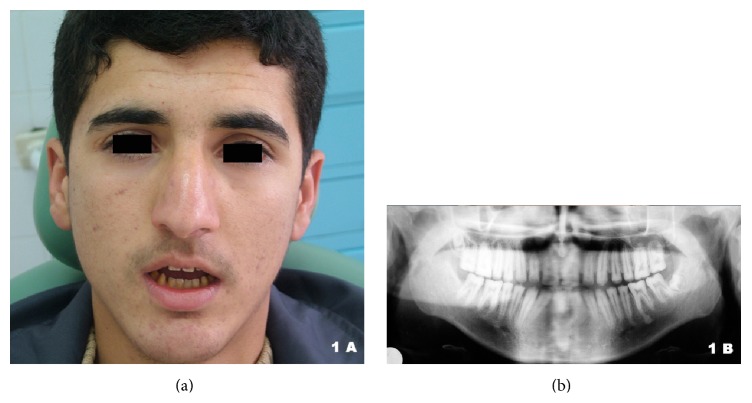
Facial appearance (a) and panoramic radiograph (b) of the patient before treatment.
